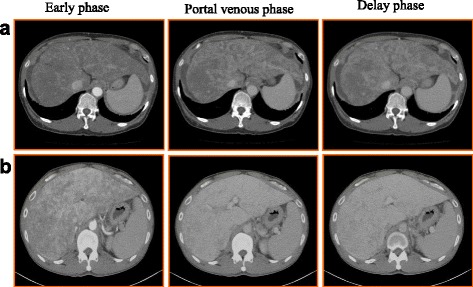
a Case 1. b Case 2. Computed tomography (CT) imaging revealed hepatosplenomegaly and the contrast effect of the liver was blocky. These findings may be an indicator of angiosarcoma
